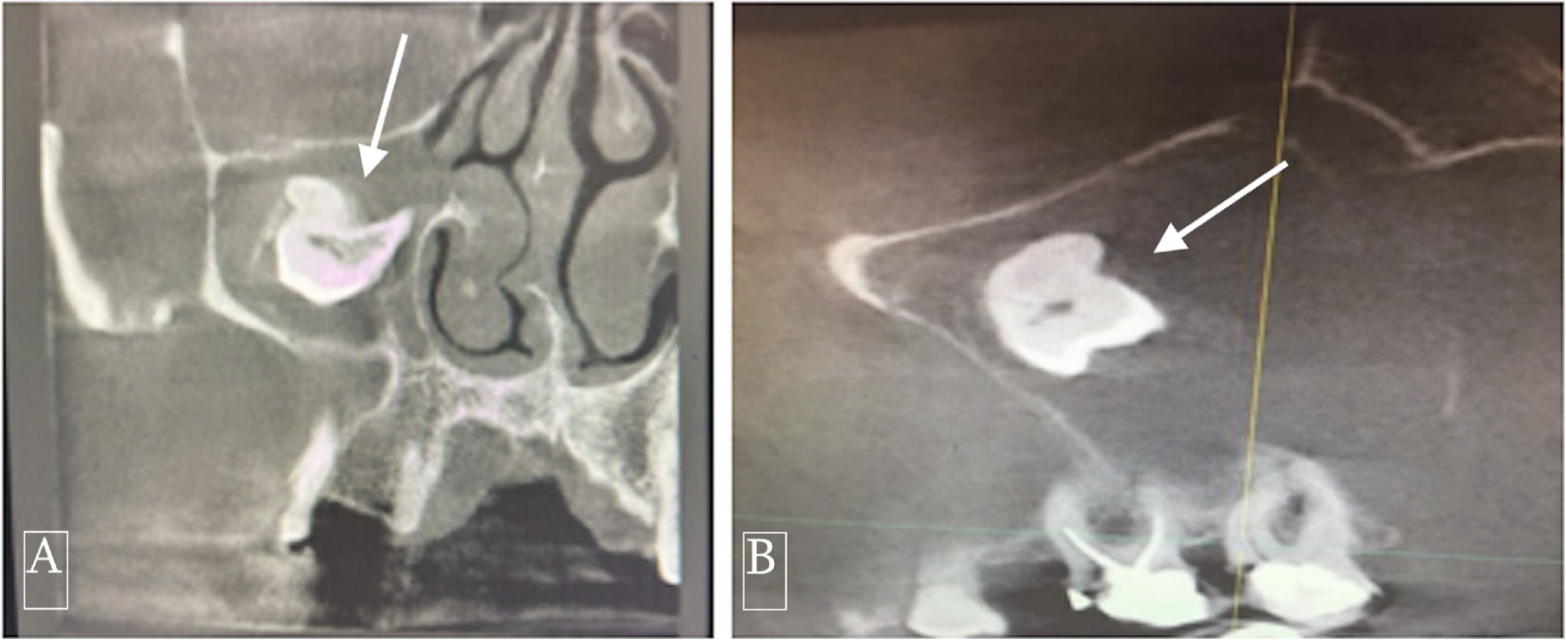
Preoperative cone beam computer tomography images (the best visualizations) showing ectopic tooth (white arrows) in the right maxillary sinus. (A) Coronal view. (B) Sagittal view.
